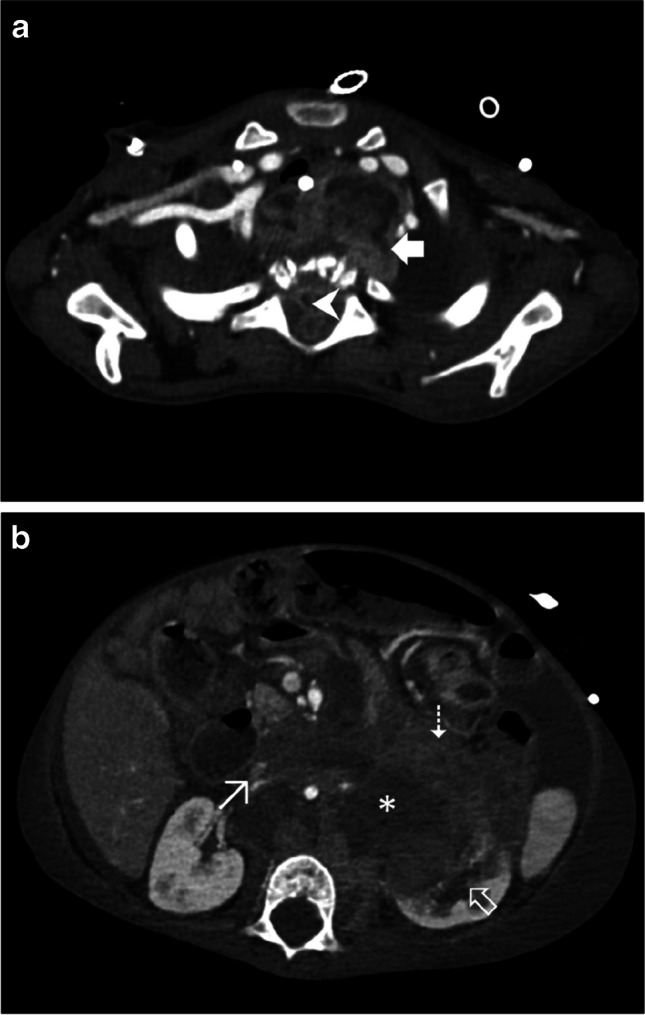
A 1-year-old boy with a history of an extensive thoracic and abdominal neuroblastoma. a Axial computed tomography (CT), post-contrast, following a split-bolus protocol, upper-thoracic image (soft tissue window) with tumoral mass (*solid bold arrow*) in the mediastinum invading the spine canal (*arrowhead*). b Axial CT, post-contrast, following a split-bolus protocol, upper-abdominal image (soft tissue window) with mass (*asterisk*) in the retroperitoneum invading the left kidney (*outlined bold arrow*) and the pancreatic tail (*dashed arrow*). Note the laminated aspect of the inferior vena cava (*thin arrow*)
